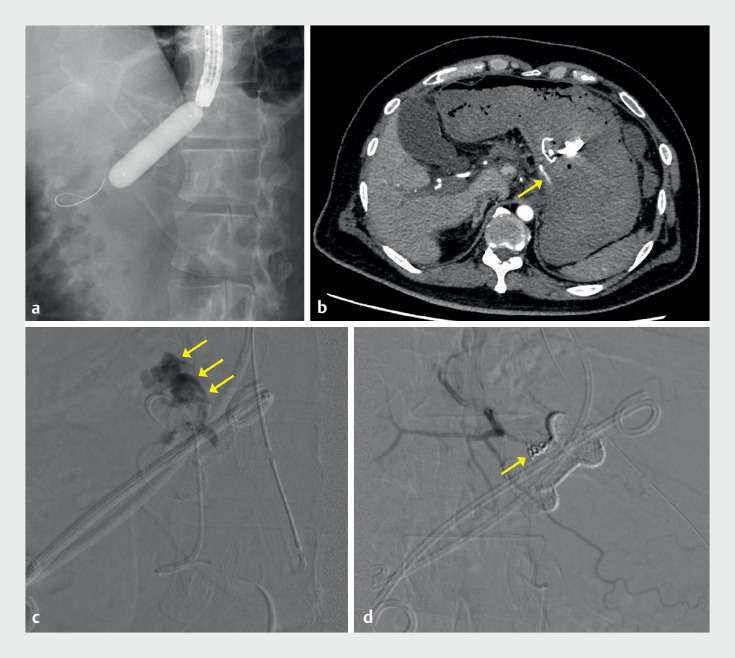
Severe bleeding associated with endoscopic ultrasound-guided placement of a lumen-apposing metal stent (LAMS) for walled-off necrosis (WON).
